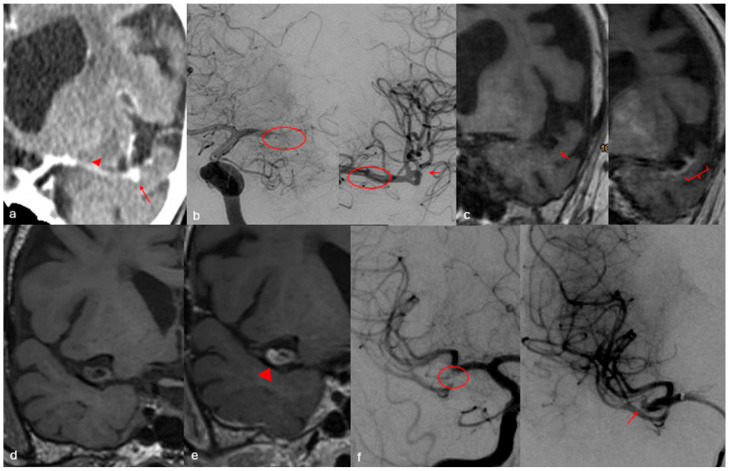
Two cases with parent vessel occlusion. The first case is shown in the upper row. (a) Late-phase CT with contrast shows retrograde filling of a left MCA bifurcation aneurysm (red arrow) and a filling defect of the proximal M1 segment (red arrowhead). (b) DSA of the left ICA shows an M1 occlusion and complete recanalization after successful mechanical thrombectomy (red circle). The LMCA bifurcation aneurysm is seen after recanalization (red arrow). (c) MRI with vessel wall imaging shows no contrast enhancement of the aneurysm wall (red arrow). Cortical enhancement of the left temporal lobe due to subacute ischemic lesions is seen (red bracket). In this case it is improbable that the aneurysmatic lesion was responsible for the stroke. The second case is shown in the lower row where a right MCA bifurcation aneurysm shows intense aneurysm wall enhancement on MRI vessel wall imaging (d,e) (red arrowhead). T1 sequence without (d) and with contrast (e) is shown. (f) DSA of the right MCA shows occlusion at the origin of the inferior trunk (red circle) with complete recanalization and appearance of the right MCA bifurcation aneurysm (red arrow) after successful aspiration of the clot. In this case the thrombosed aneurysm was regarded as the probable cause of stroke and was coiled at a later date.
